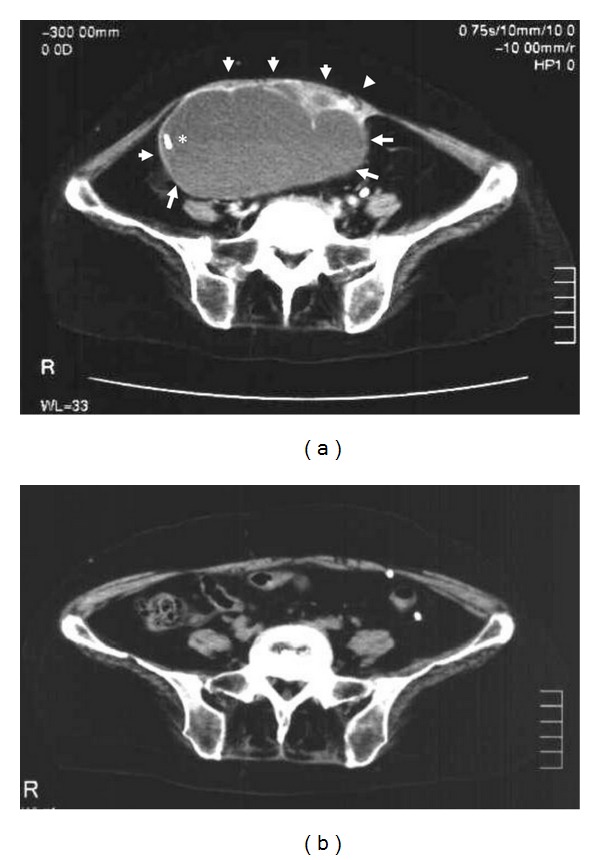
Preoperative abdominal CT scan image with contrast medium (a) showing a huge multilocular cyst-like enhanced mass (arrowheads) in the peritoneal space around the distal tip of the VP shunt tube (∗). An abdominal CT image that was performed 2 months after the laparotomy (b) indicating no recurrence of the pseudocyst.
